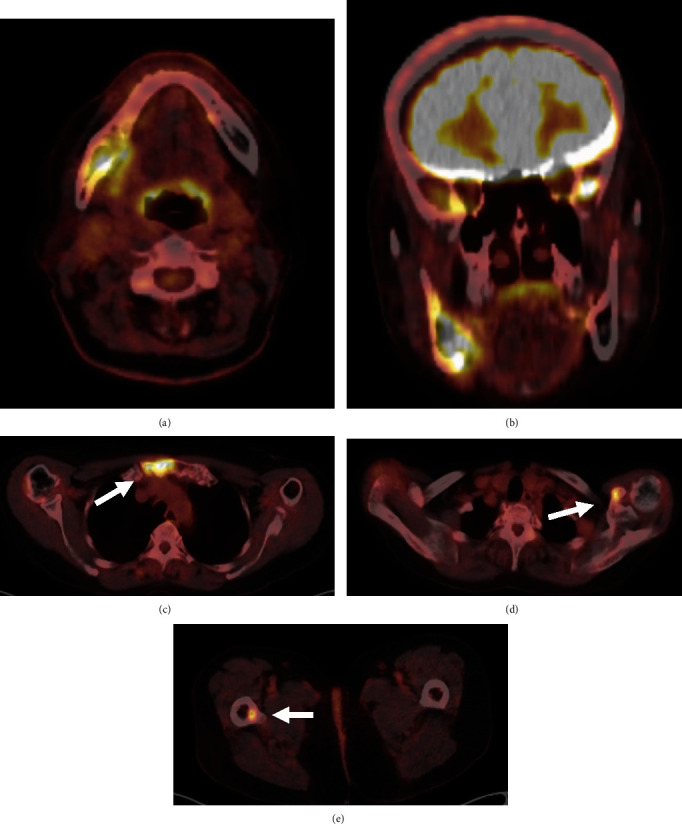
PET–CT imaging. PET imaging showed increased uptake in (a) and (b) right side of mandible, (c) left coracoid process of scapula, (d) sternum, and (e) right lesser trochanter of thigh bone.
